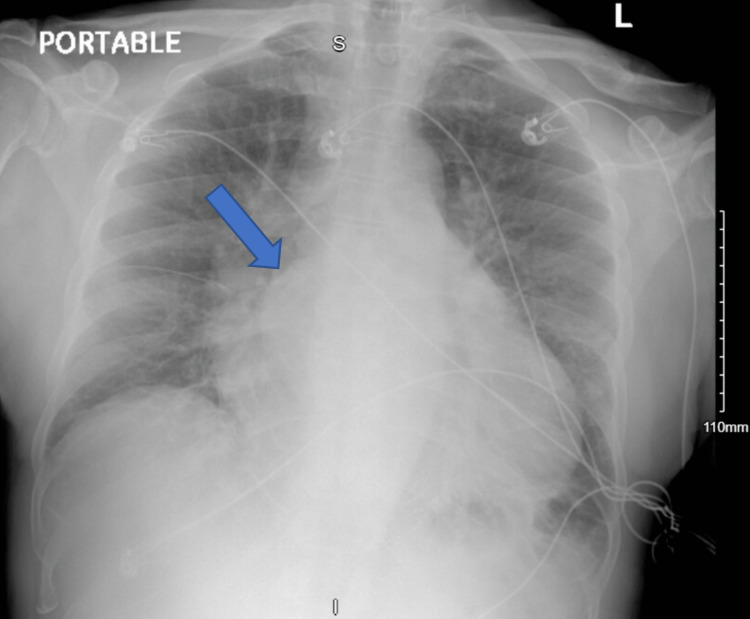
Chest X-ray revealing interstitial pulmonary edema and enlarged subcarinal opacity (arrow) suggesting right atrial enlargement.
